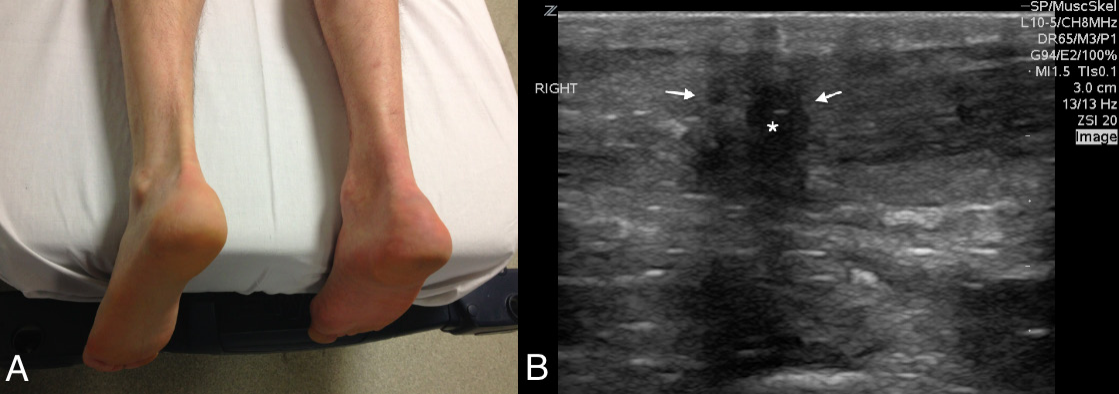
(A) Comparison of both posterior ankles in patient with right posterior Achilles tendon rupture. (B) Longitudinal ultrasound image of the area of pain to the posterior right ankle, noting retracted ends of the Achilles tendon (arrows) and hematoma in-between (*).
